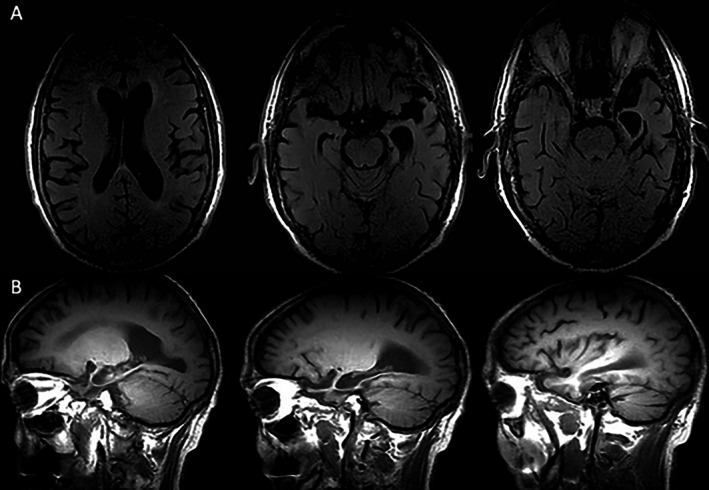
Brain MRI showing atrophy of the left temporal lobe, including both medial structures with amygdala‐hippocampal involvement and anterior regions. (A) Fluid‐attenuated inversion recovery (FLAIR) sequence, axial plane. (B) T1‐weighted MRI scan, sagittal plane.
